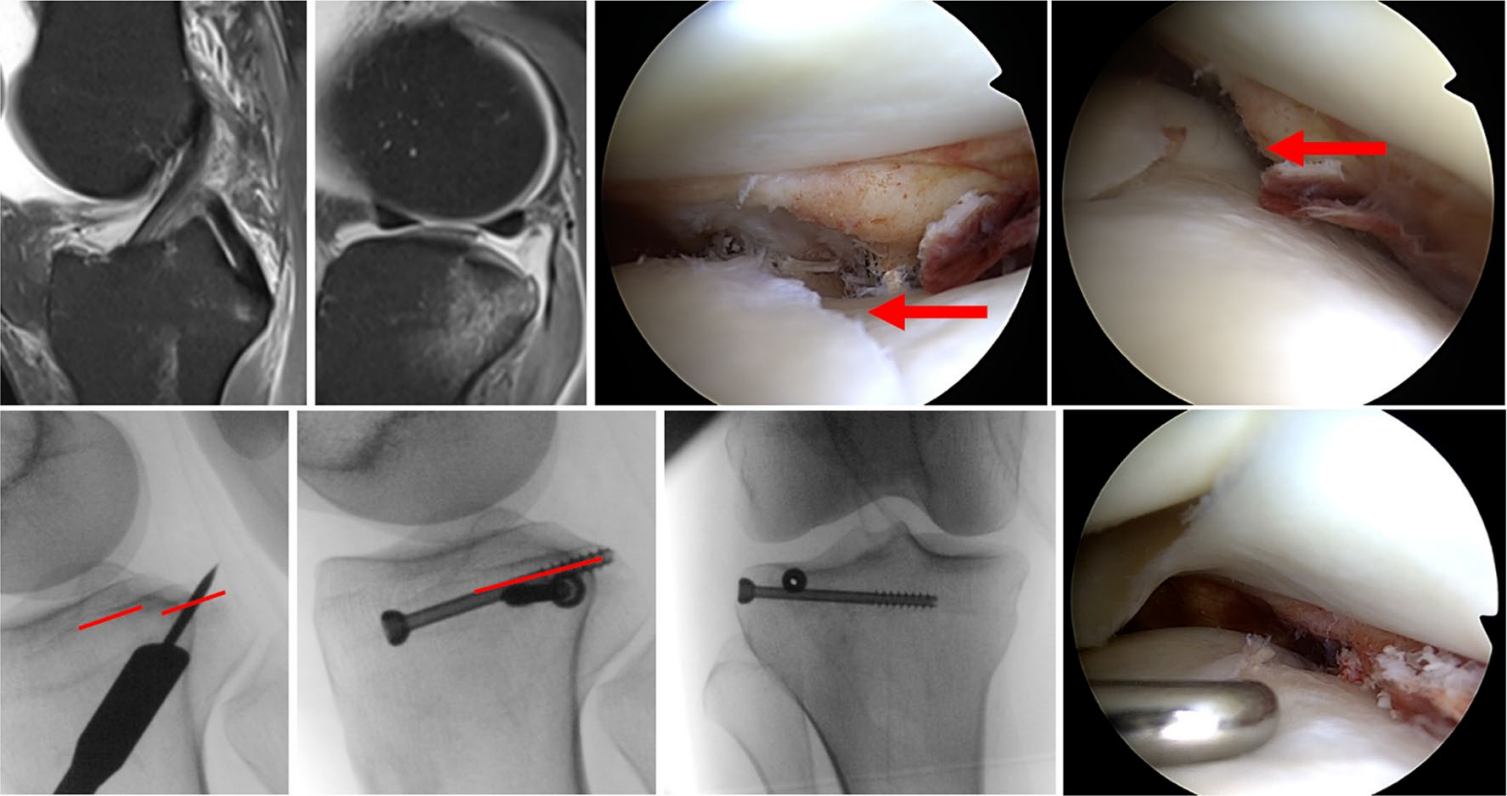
A 31-year-old female after skiing trauma with negative Lachman and intact ACL, but positive pivot shift due to isolated displaced PLIF type 2c [Menzdorf et al.[22]] or type IIIB [Bernholt et al. [21]]. MRI and arthroscopic findings (red arrows) show the PLIF of more than 2 mm depression and more importantly the complete width of the posterior horn of the lateral meniscus (upper row). Intraoperative findings demonstrate arthroscopic anatomic reduction and minimally invasive fixation as well as improved bony support of the posterior horn of the lateral meniscus (ARIF, lower row)
